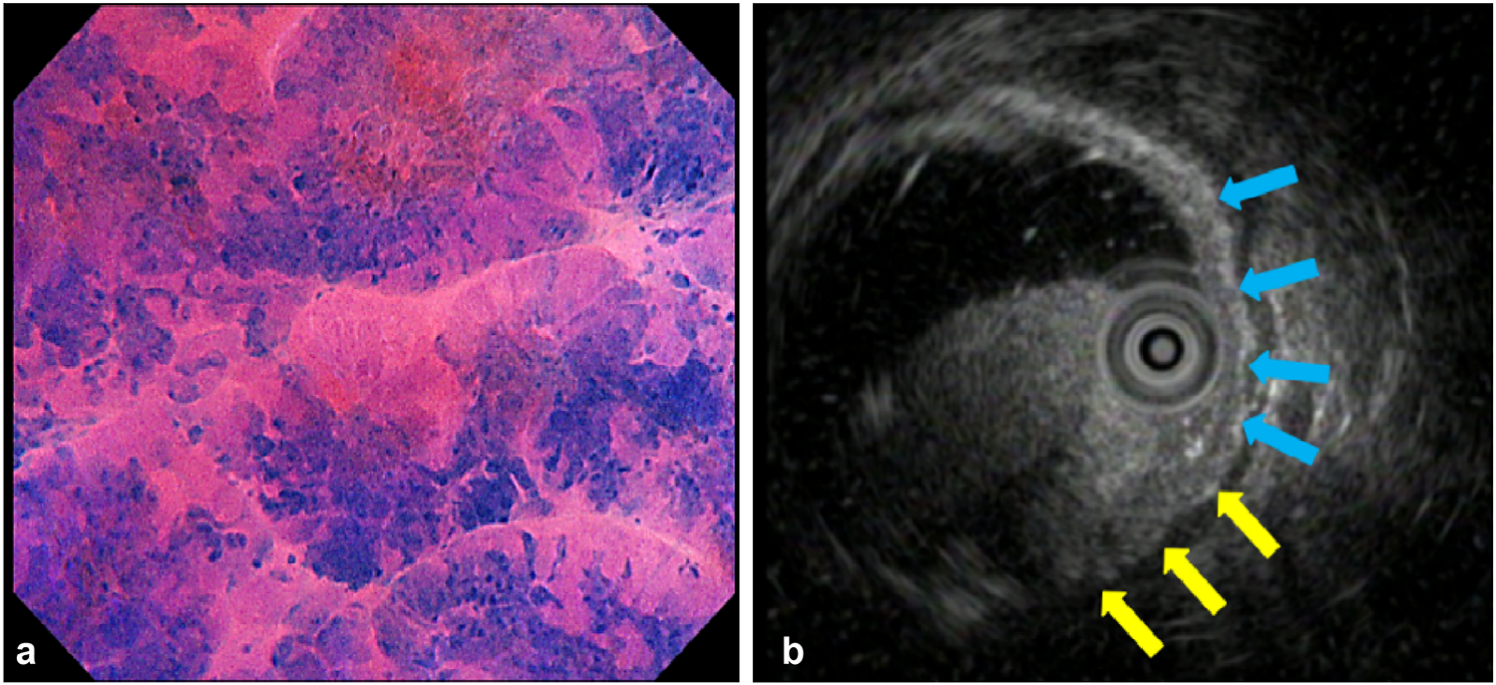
(a) An ultra‐magnified endoscopic image classified as EC3a; (b) An endoscopic ultrasonography image. The area indicated by the blue arrow can be traced in the third layer (submucosal layer), but it appears to be interrupted (tumor invasion) at the area marked by the yellow arrow.
